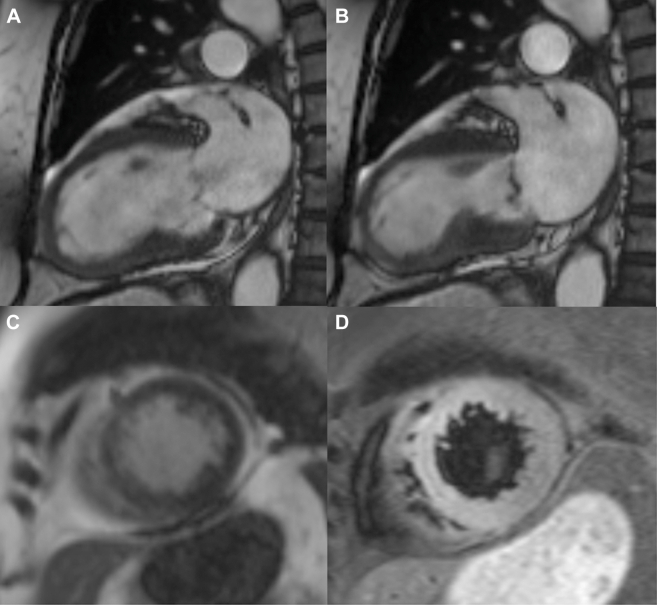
Contrast-Enhanced CMR on Day 7 After Takotsubo Syndrome Onset (А) Cine image, 2-chamber slice, diastolic phase. (В) Cine image, 2-chamber slice, systolic phase. Hypokinesis of the apical LV segments is observed. (С) CMR with delayed contrast enhancement, LV short axis at the level of apical segments shows no gadolinium uptake in the myocardium. (D) Т2-weighed image. LV short axis at the level of apical segments. Edema of the interventricular septum extending to the LV anterior wall. CMR = cardiac magnetic resonance imaging; LV = left ventricle.
